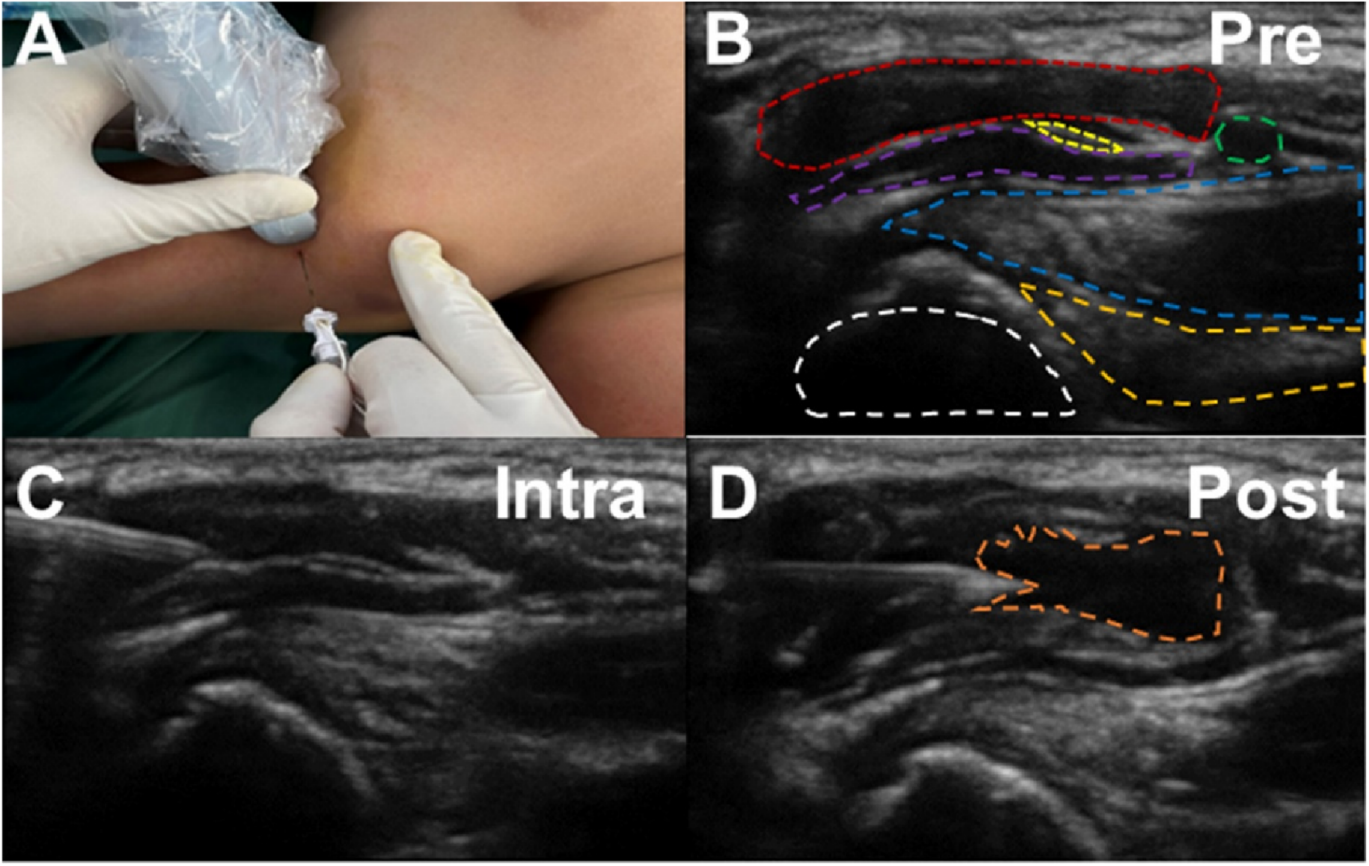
The representative patient's medical images of ultrasound-guided coracobrachialis plane musculocutaneous nerve block. (A) Pre-puncture ultrasound image. White: Humerus; Dark yellow: Teres major; Blue: Latissimus dorsi; Purple: Coracobrachialis; Yellow: Musculocutaneous nerve; Red: Biceps; Green: Axillary artery. (B) Intra-puncture ultrasound image: The puncture reaches the fascial layer. (C) Intra-puncture ultrasound image: The fascia is stretched by the injection. (D) Post-injection ultrasound image. Orange: local anesthetic agent injected.
